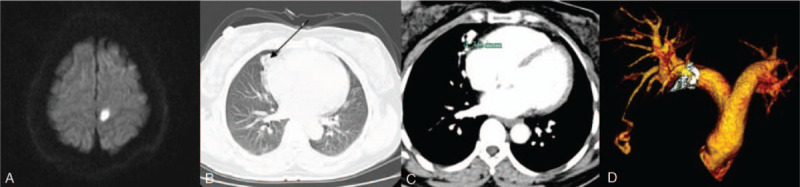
Magnetic resonance imaging detects an acute stroke in left temporal parietal lobe and right parietal lobe (A). Unenhanced chest computed tomograph scan cross-section depicts a chordae shadow in right middle lobe (B, black arrow). Enhanced pulmonary computed tomographic angiography re-confirms a PAVF with a 4.3-mm feeding artery in diameter located in the consistent position as showed on the unenhanced computed tomograph scan (C). Reconstructed image demonstrates its tortuous, spiral tridimensional morphology (D).
